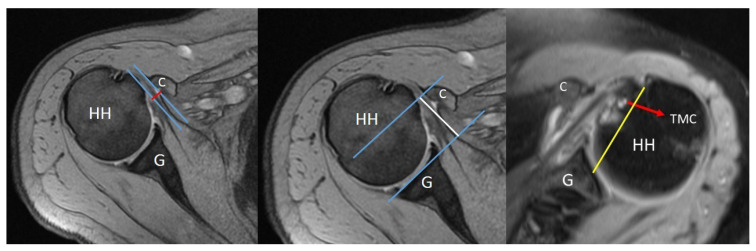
Coracohumeral distance (CHDax-red line), coracoid overlap (CO-white line) measurement, and tuberculum minus cysts (TMC-red arrow) on axial sections of magnetic resonance imaging scans.
